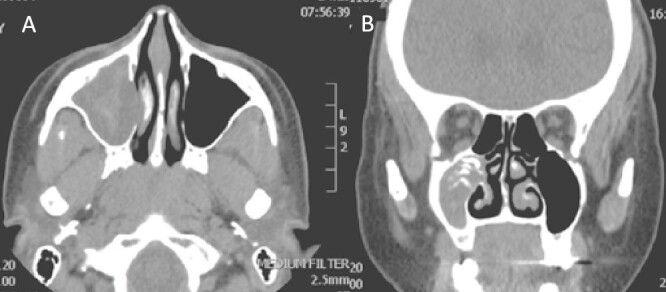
Axial (A) and coronal (B) images of a nonenhanced CT scan of the paranasal sinuses showing right maxillary sinus complete heterogenous opacification.
